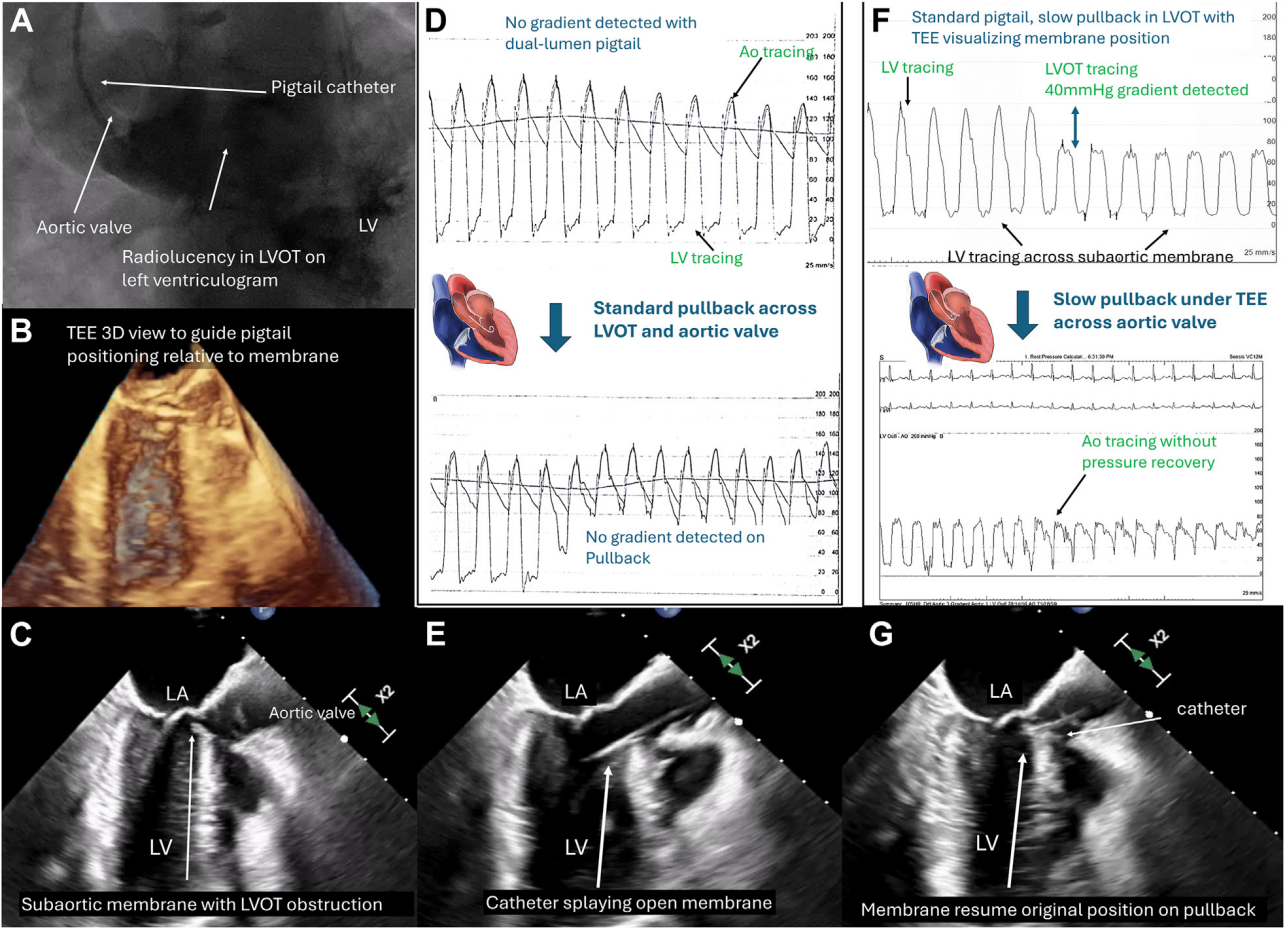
(A-C) Appearance of subaortic membrane on left ventriculogram and transesophageal echocardiography (TEE). (D-E) No gradient detected with a dual-lumen pigtail catheter as the subaortic membrane is splayed open as seen on TEE. (F-G) Slow pullback on TEE unmasks the pressure gradient as membrane resumes original position. LA, left atrium; LV, left ventricle; LVOT, left ventricular outflow tract.
